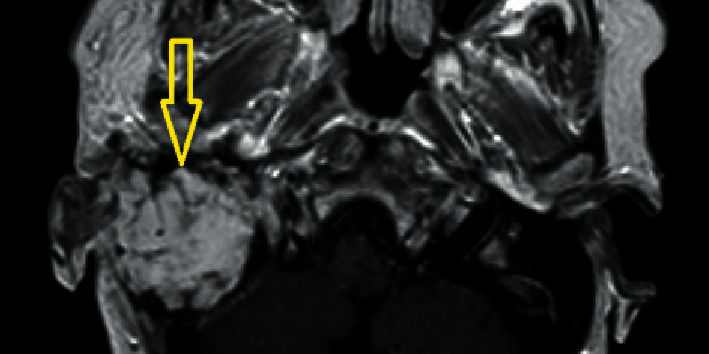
Axial T1-weighted MRI with gadolinium revealed obliteration with abdominal fat (yellow arrow) without any residual tumor 16 months after the extended temporal bone resection.
